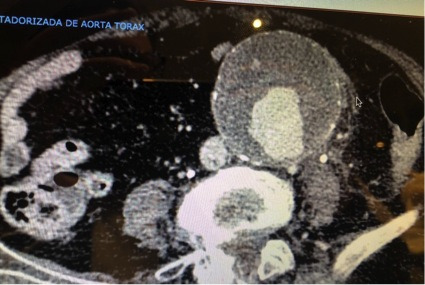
Axial Angio-CT scan showing ruptured aorta in case 1.
